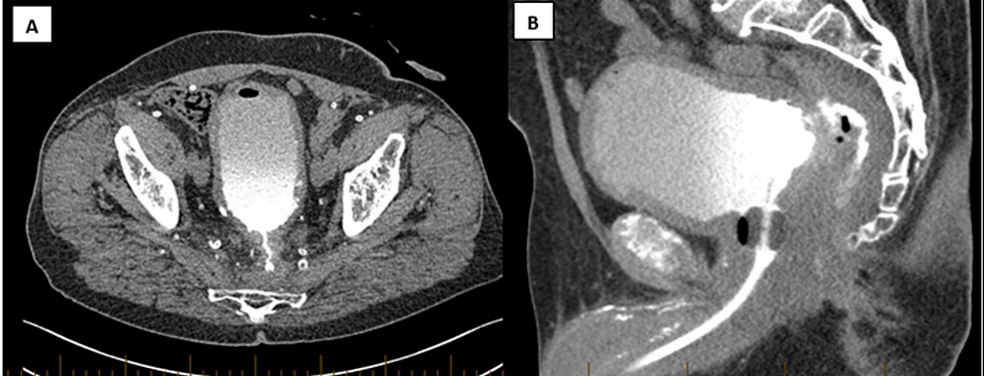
CT Cystogram (A) CT cystogram demonstrating a VCF arising from the posterior aspect of the bladder wall. It traverses the presacral space posteriorly and then inferiorly towards the perineum. (B) CT cystogram demonstrating the VCF arising from the mid-line of the posterior bladder wall with parietal irregularity.
